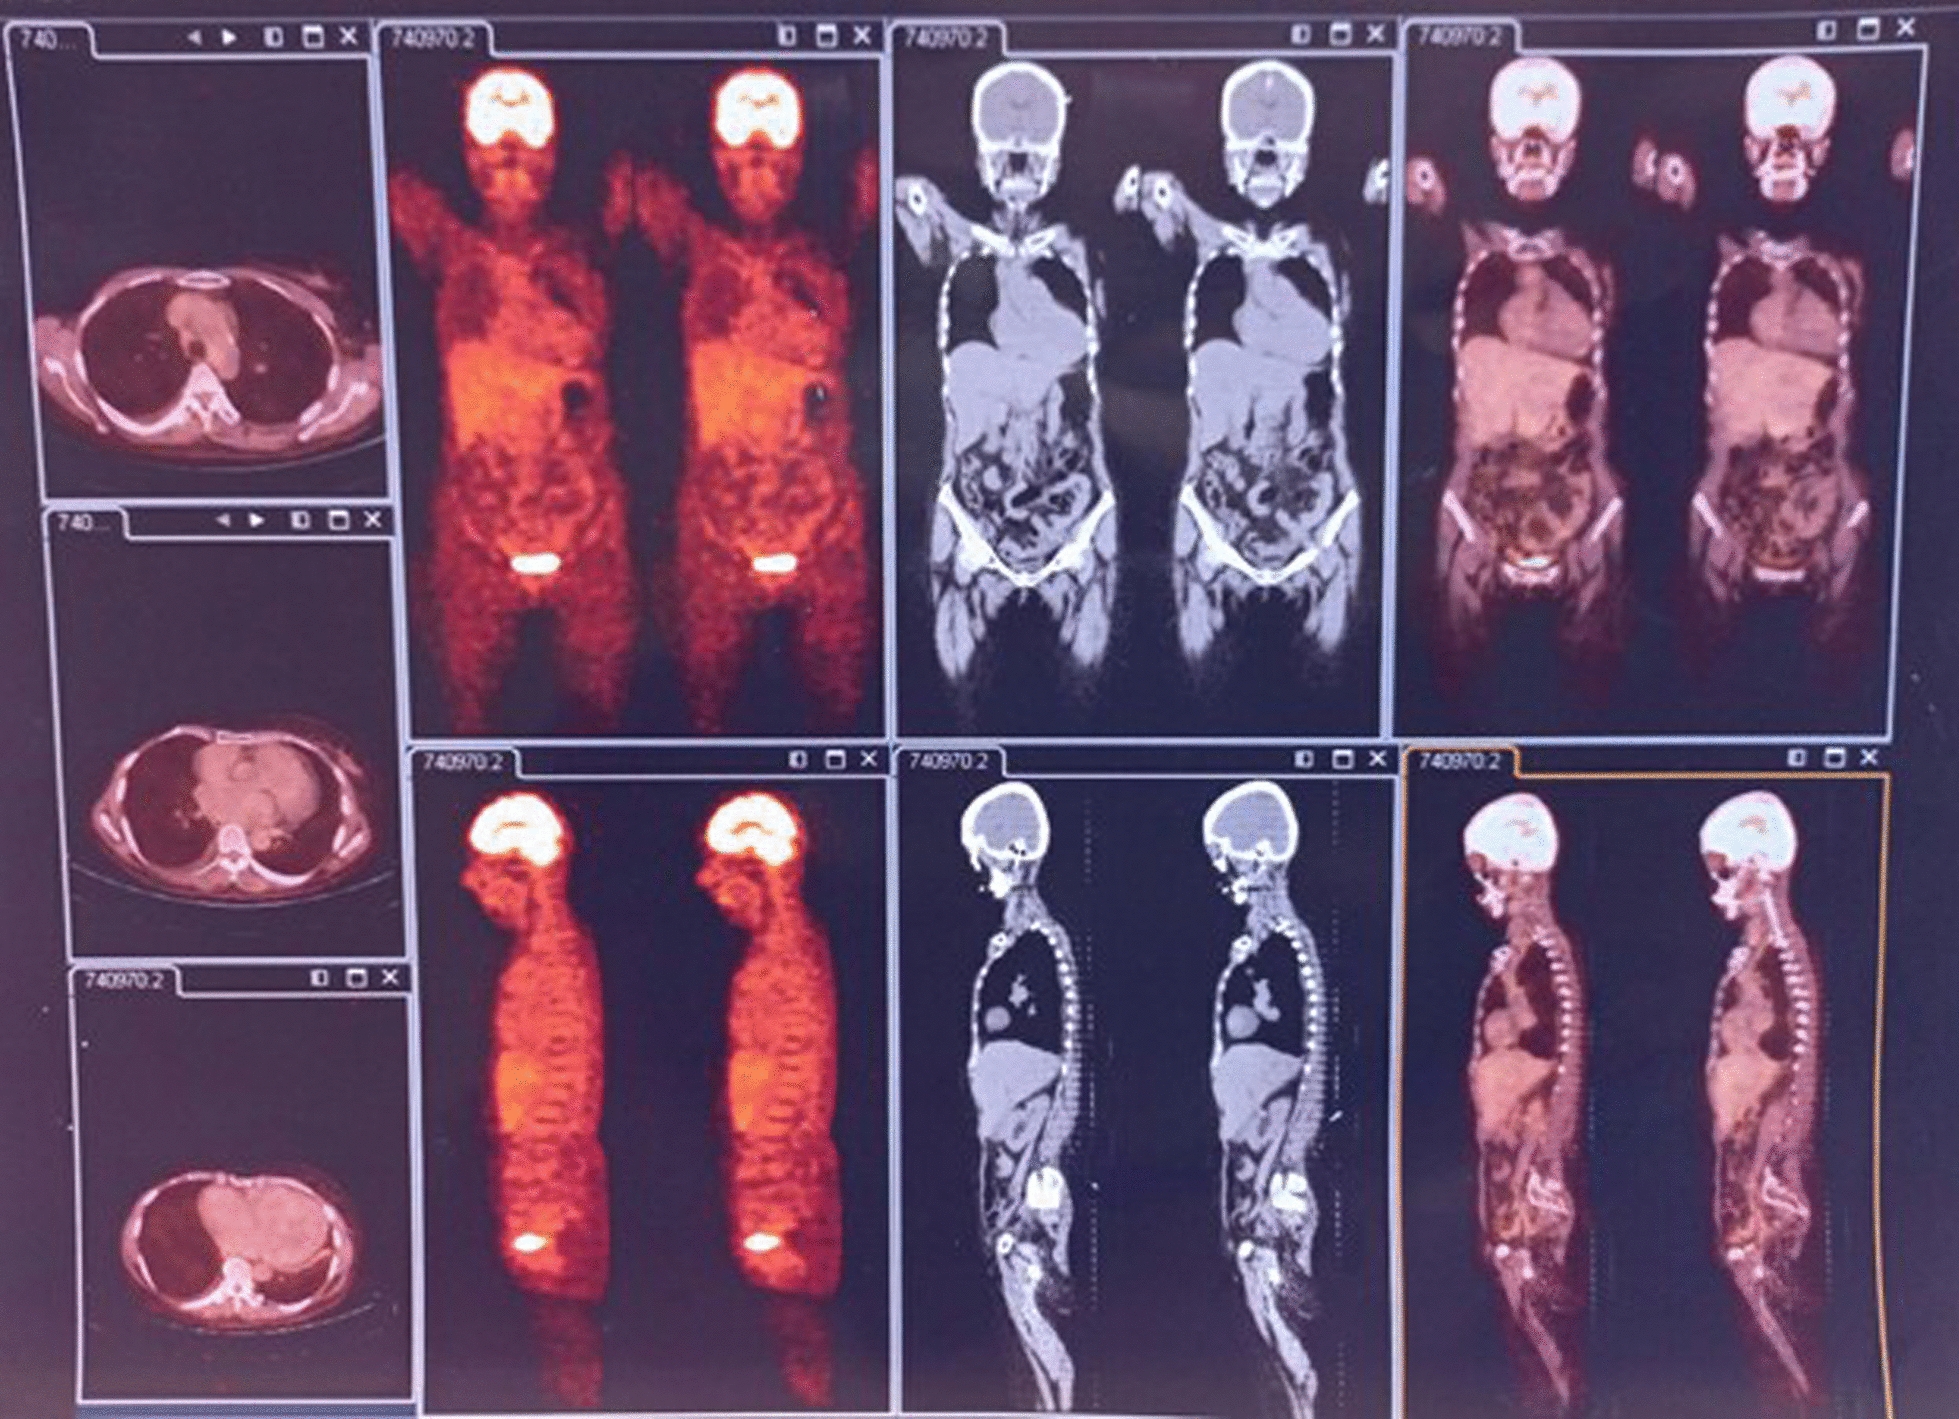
A positron emission tomography-computed tomography in October 2023 demonstrated a complete response to endocrine monotherapy
